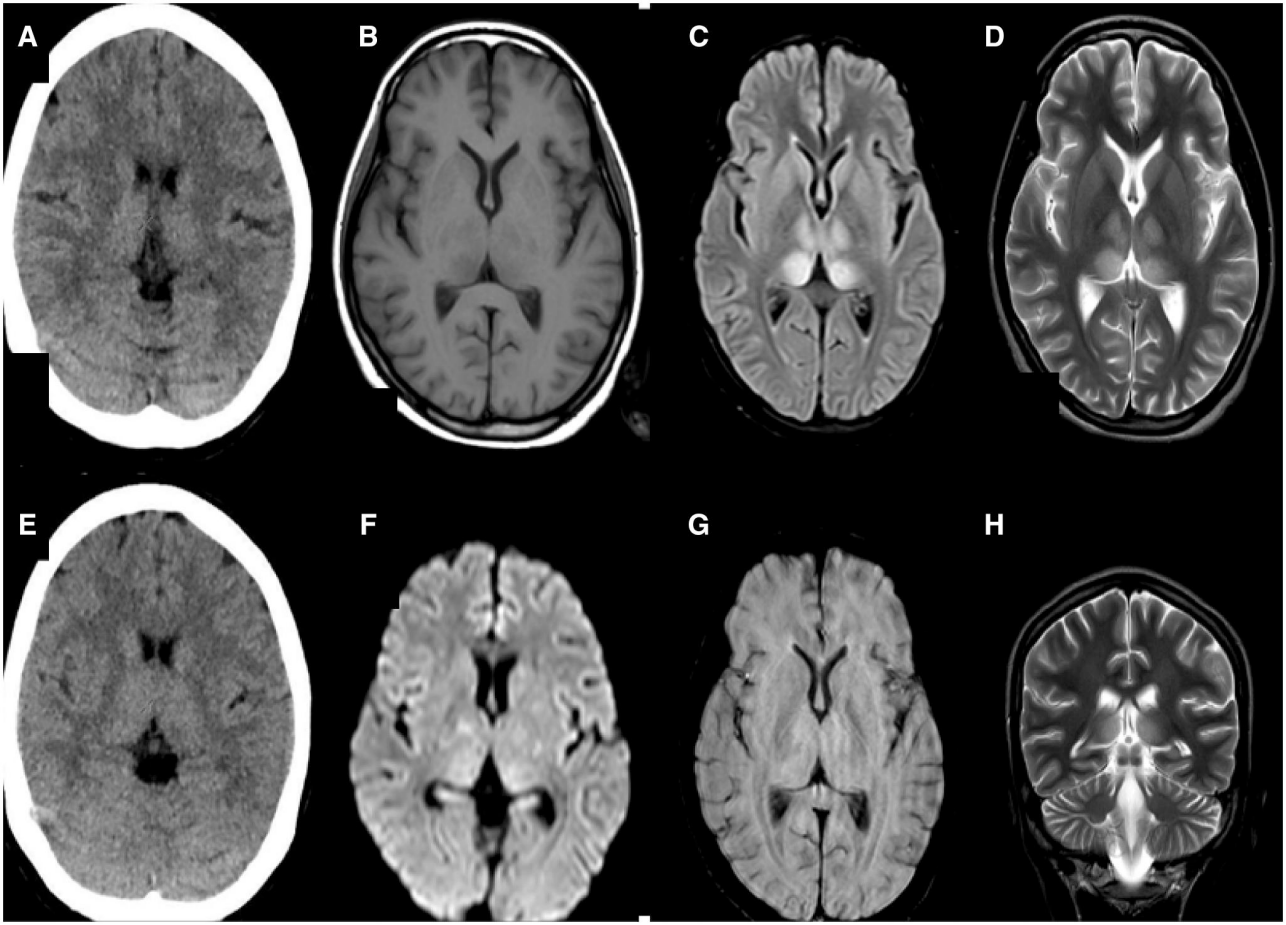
Axial CT showed subtle symmetrical hyperdensity in bilateral thalami. No hemorrhagic component seen (A and E). Subtle T1 hypointensity (B) in bilateral thalami. The corresponding lesion appears hyperintense T2/FLAIR hyperintense (C, D). Subtle diffusion restriction seen with high signal on DWI (F). No hemorrhagic component seen in SWI images (G).
